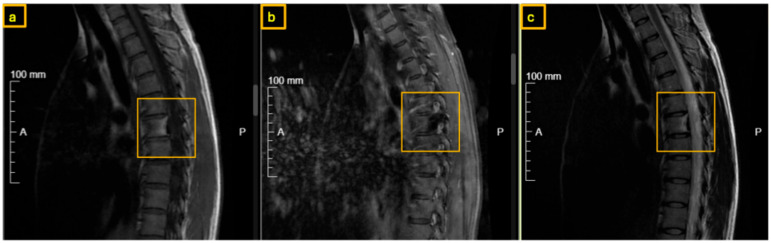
Preoperative MRI of the thoracic spine. The yellow square indicates the nidus at the root of the right T7 pedicle: (a) T1-weighted, (b) T1-weighted contrast-enhanced, and (c) T2-weighted sagittal views.
